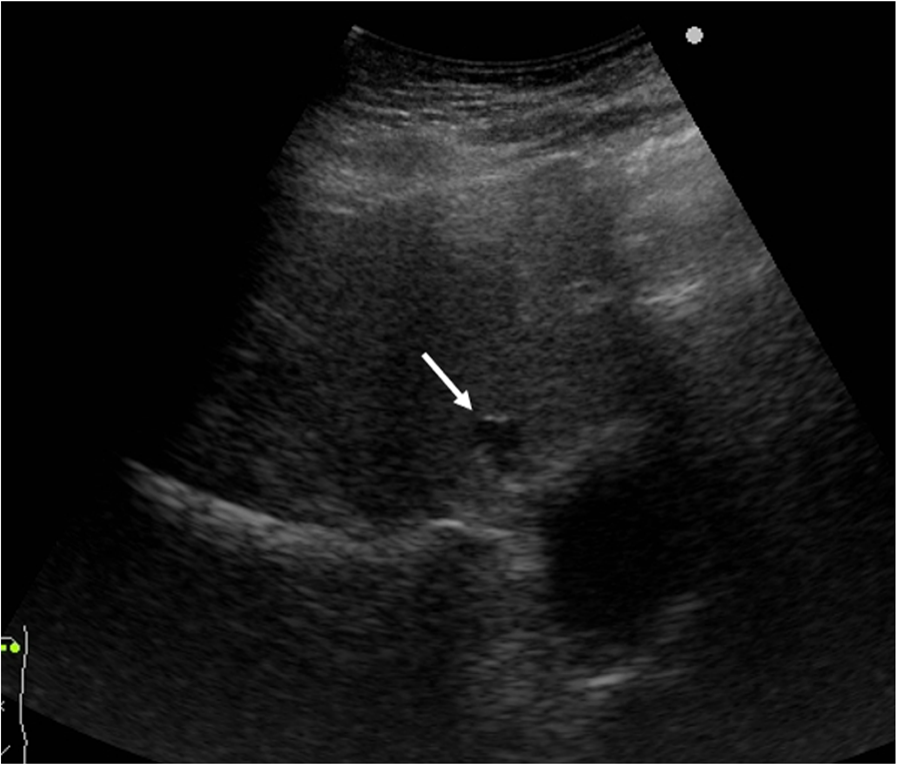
Postoperative abdominal ultrasonography findings. The abscess (white arrow) became smaller
